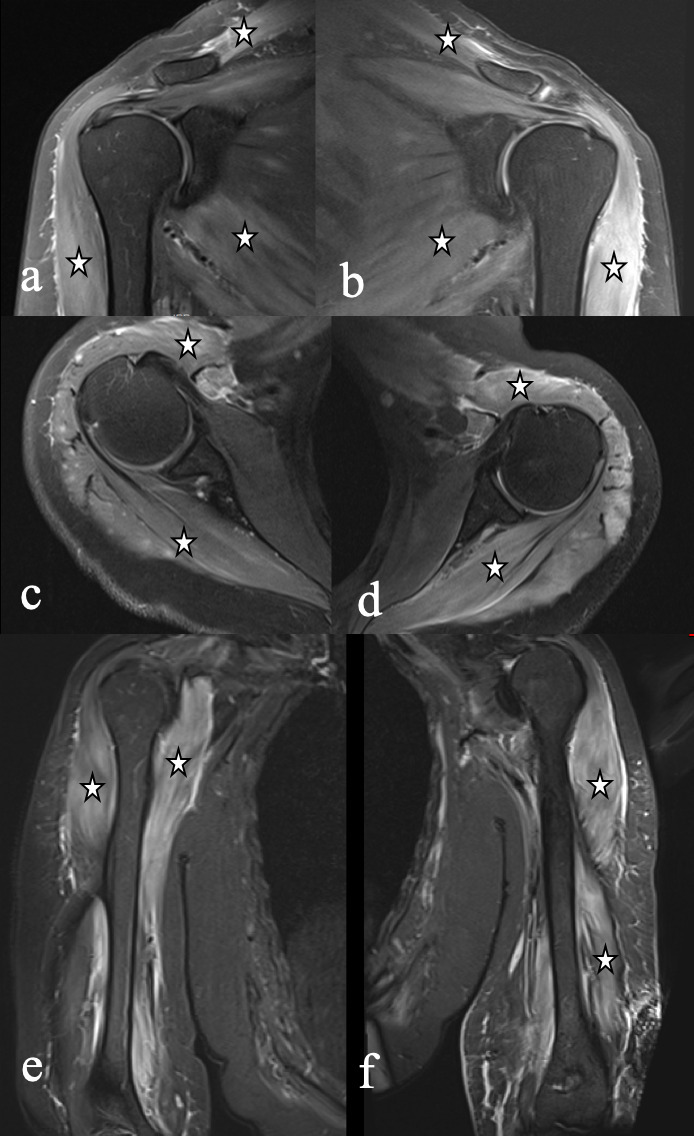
Right (a) and left (b) coronal T2 fat-saturated shoulder MR images demonstrating trapezius, deltoid, and rotator cuff muscle edema (white stars). Similar findings were observed in axial (c, d) coronal T2 fat-saturated shoulder MR images and coronal (e, f) arm MR imaging involving also biceps and triceps muscles.
